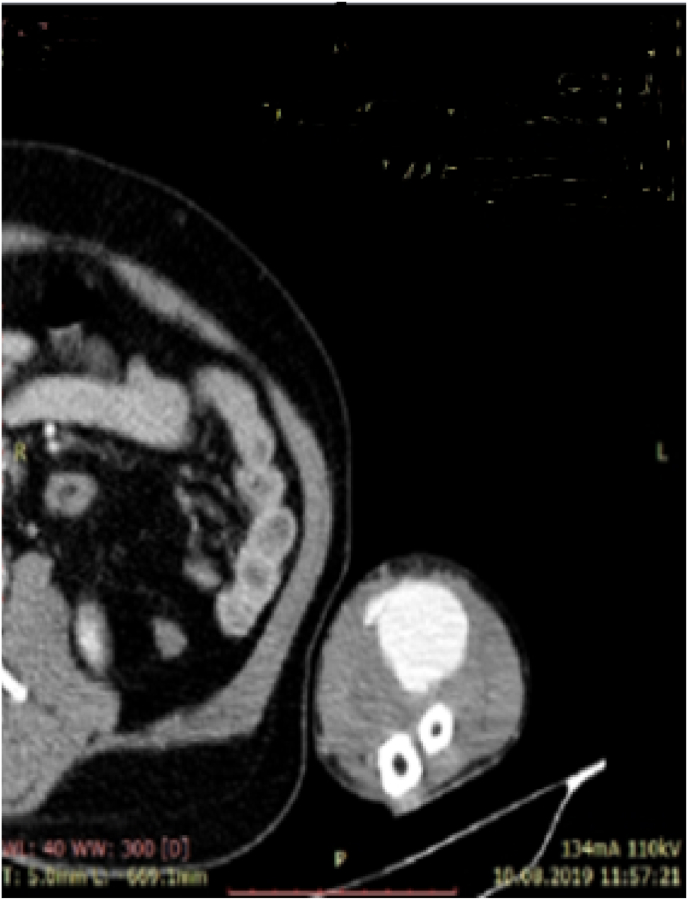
Computed tomography angiography on transverse view Showing Proximal Radial artery pseudoaneurysm.
